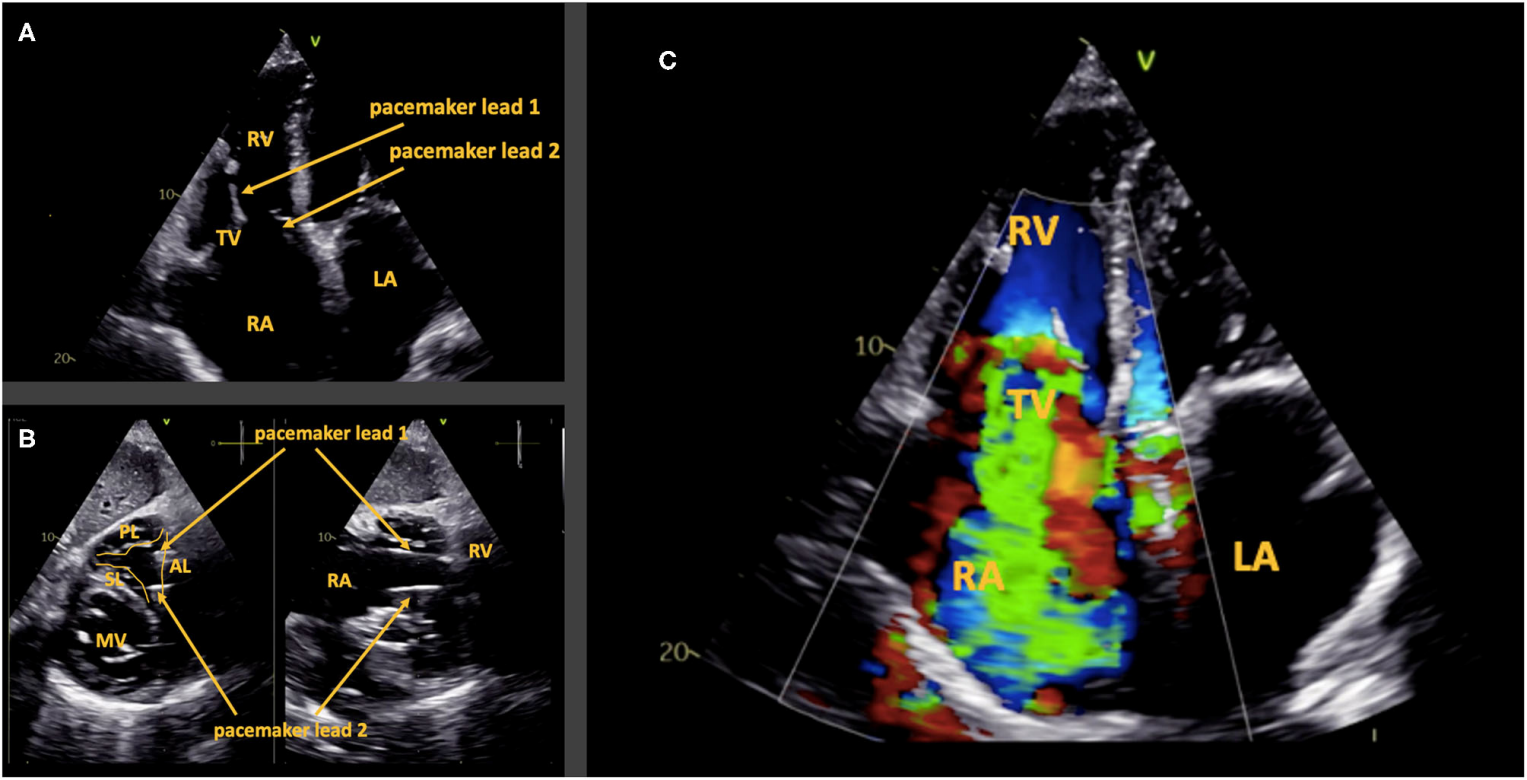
Case 3: Anterior leaflet impingement by a pacemaker lead. Two trans-tricuspid pacemaker leads are present (A). The transthoracic subcostal en face view of the tricuspid valve shows one lead in the antero-posterior commissure and the other in the antero-septal commissure (B). Leaflet impingement of the anterior leaflet causes “torrential” tricuspid regurgitation (C). AL, anterior leaflet; PL, posterior leaflet; SL, septal leaflet; RA, right atrium; RV, right ventricle; LA, left atrium; TV, tricuspid valve; MV, mitral valve.
